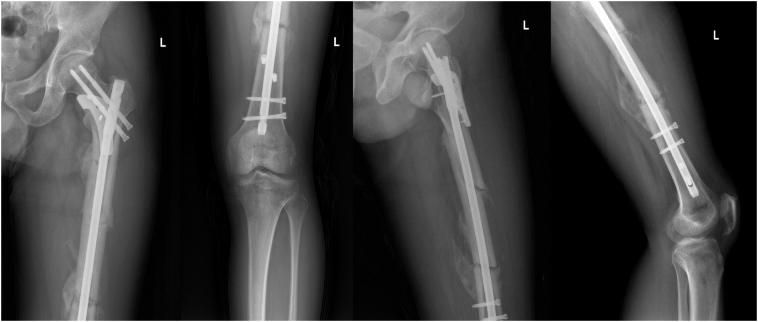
Radiographs were taken three months after surgery showed fracture healing.
